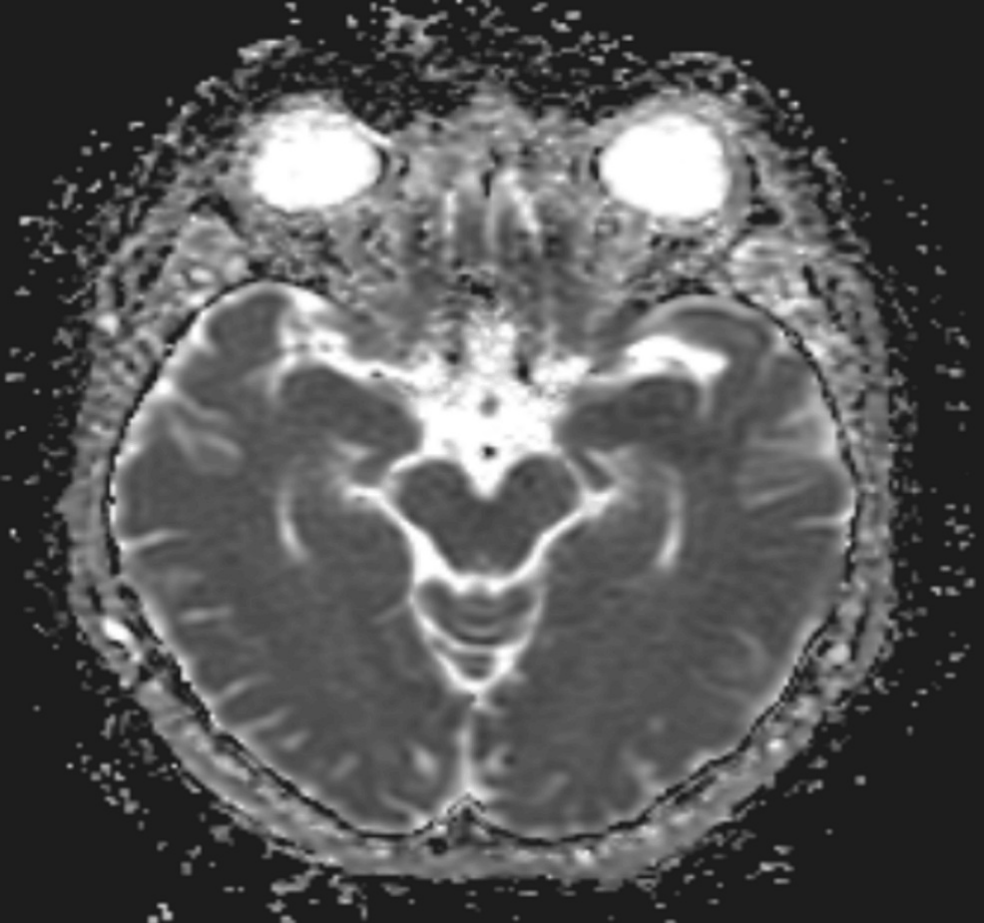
Apparent diffusion coefficient (ADC) MRI
